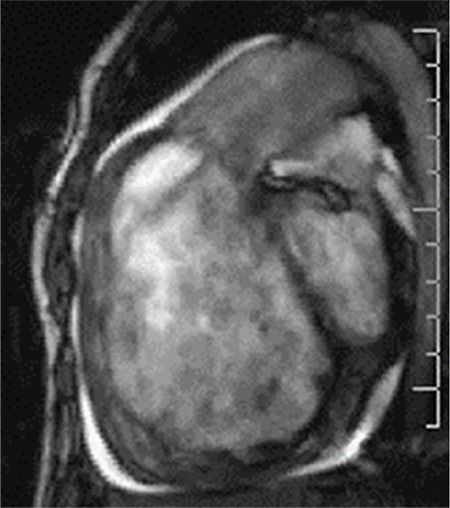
Magnetic resonance imaging of the right ventricle and pulmonary regurgitation in a patient with tetralogy repair. The right ventricle is enlarged and regurgitant jet (black arrow) is seen.
